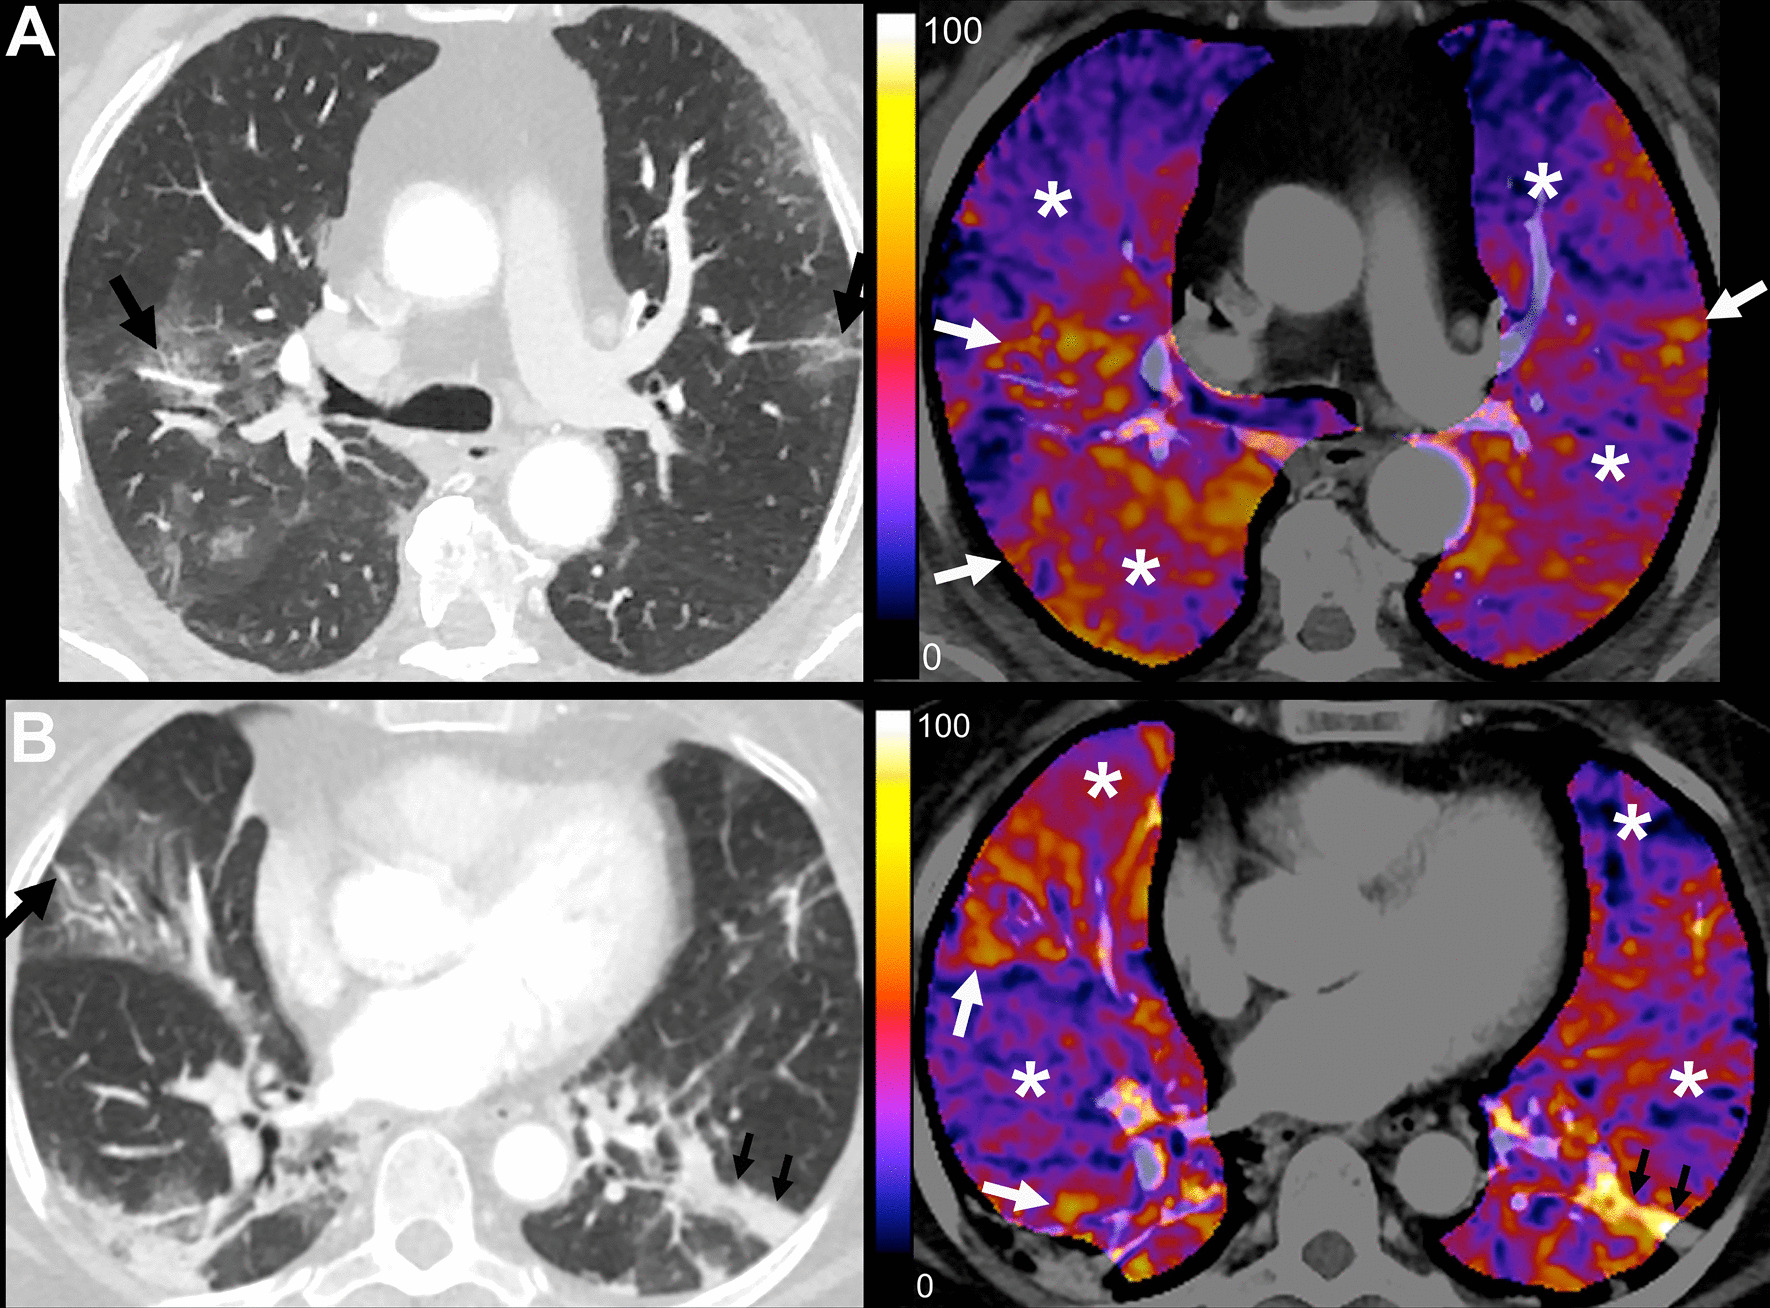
Conventional and color map sCTA images within 24 h of admission to the hospital and before administration of the first dose of the allocated intervention. A Placebo Arm. 70-year-old male patient before receiving the first dose of placebo with RT-PCR-confirmed COVID-19 at 6 days since symptom onset. Admission Charlson score was 3, A-a gradient was 75.6, and PaO_2_/FiO_2_ ratio was 322. D-dimer was 272 ng/mL. He was admitted to the ICU, managed with IMV, and died almost 7 weeks after admission. CT severity score: 9; sCTA perfusion score: 10. Moderate lung involvement with patchy ground-glass opacities in both lungs with vascular dilatation in small peripheral subsegmental pulmonary arterial branches, some of them with a varicose appearance (black arrows). Severe hypoperfusion abnormalities in apparently normal lung parenchyma (*). Some areas of ground-glass opacities show marked hyperperfusion, most likely due to vasoplegia (white arrows). B Sildenafil Arm. 64-year-old female patient before receiving the first dose of sildenafil with RT-PCR-confirmed COVID-19 at 7 days since symptom onset. Admission Charlson score was 2, A-a gradient was 109.9, and PaO_2_/FiO_2_ ratio was 207. D-dimer was 308 ng/mL. She was admitted to the ICU, managed with high flow nasal cannula, and stayed in the hospital for 14 days until discharge. CT severity score: 9; sCTA perfusion score: 10. Moderate lung involvement with patchy ground-glass opacities in both lungs and laminar atelectasis (small black arrows). There is vascular dilatation in small peripheral subsegmental pulmonary arterial branches (black arrow). Severe hypoperfusion abnormalities in apparently normal lung parenchyma (*). Some areas of ground-glass opacities show marked hyperperfusion, most likely due to vasoplegia (white arrows). Linear atelectasis shows increased perfusion in lower left lobe (small black arrows)
